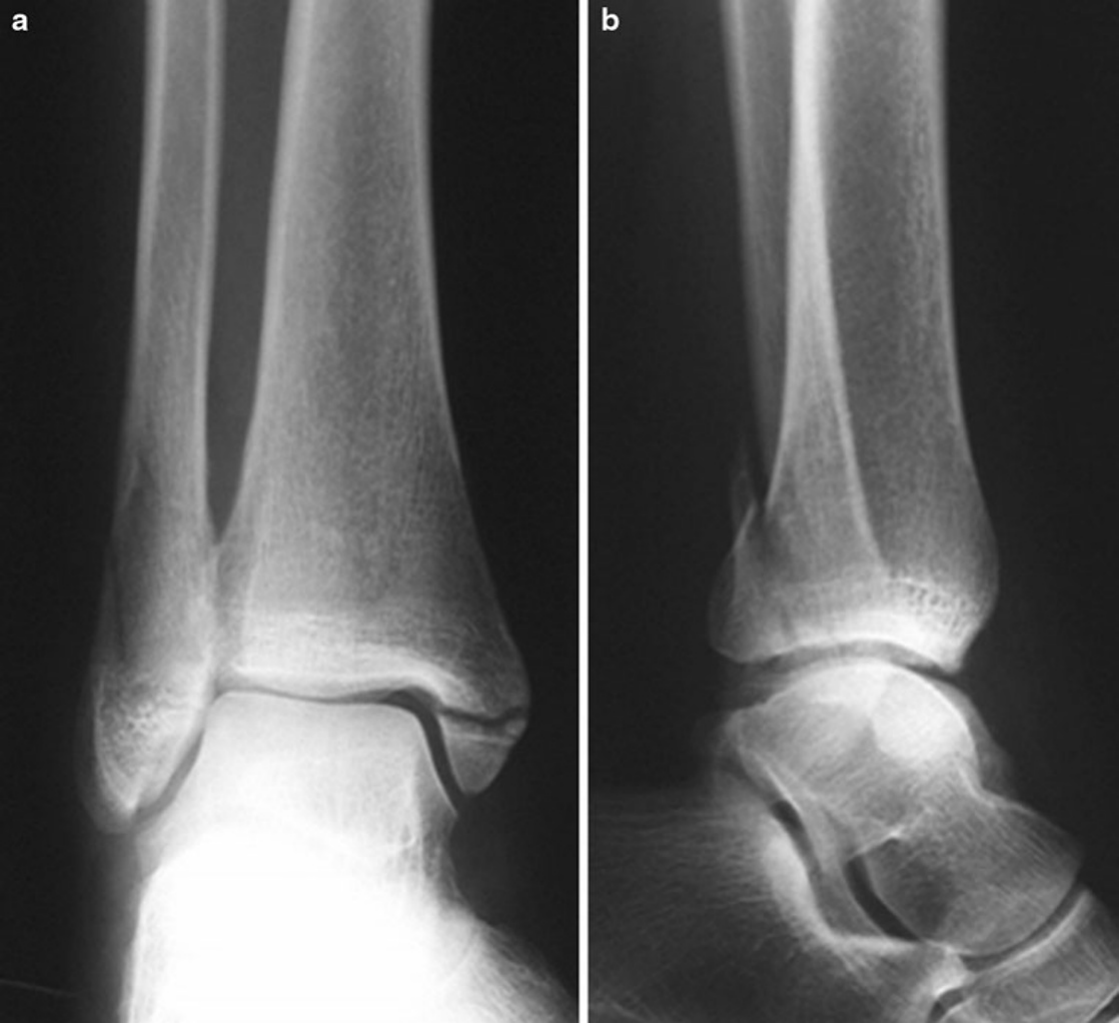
AP and lateral radiographic images of a SE-4 fracture consisting of a spiral or oblique fracture laterally and a transverse medial malleolar (avulsion) fracture
